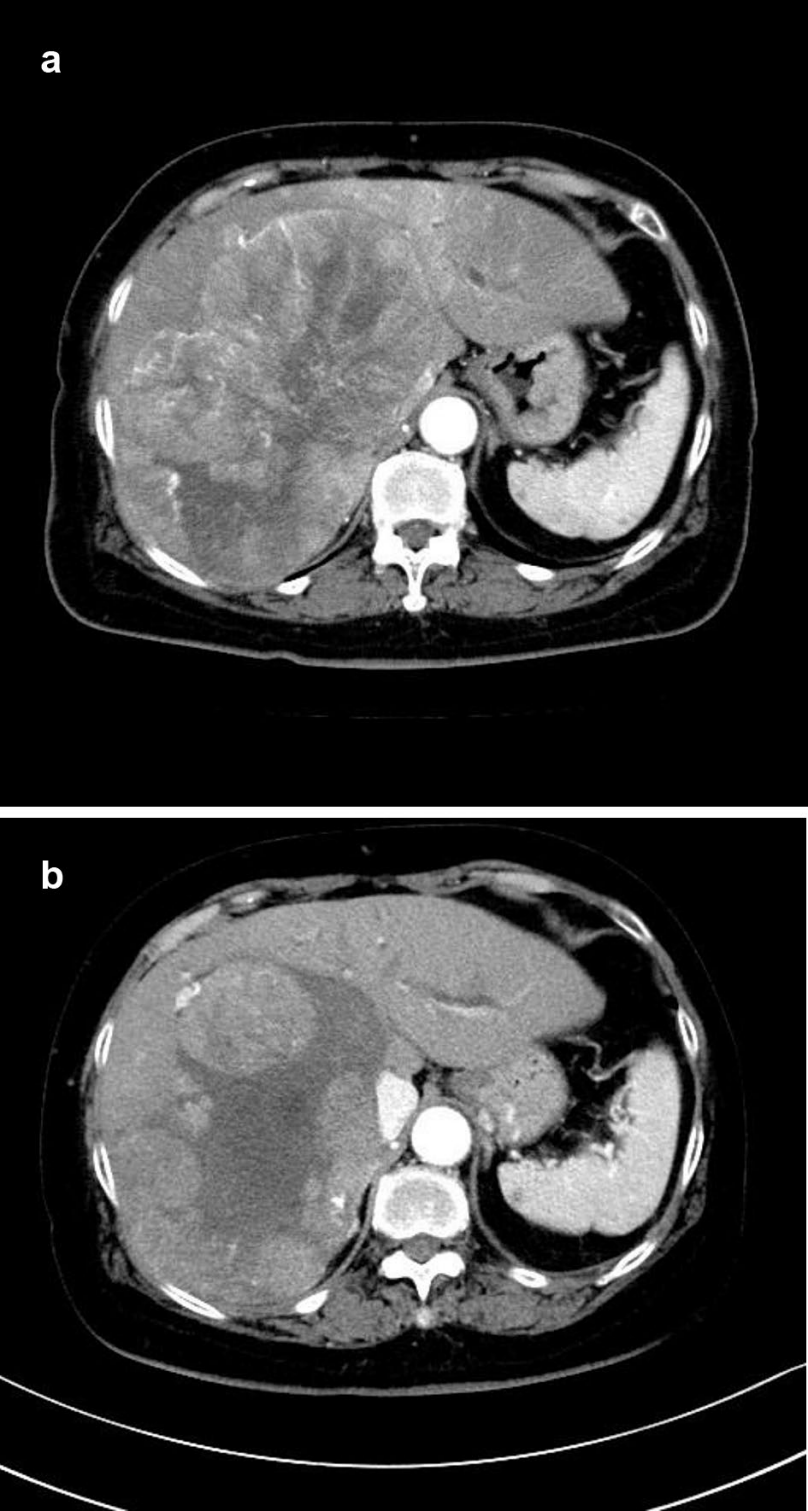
Case 3 a Contrast-enhanced CT before commencement of lenvatinib treatment. b Contrast-enhanced CT at the time of ICG retention testing
